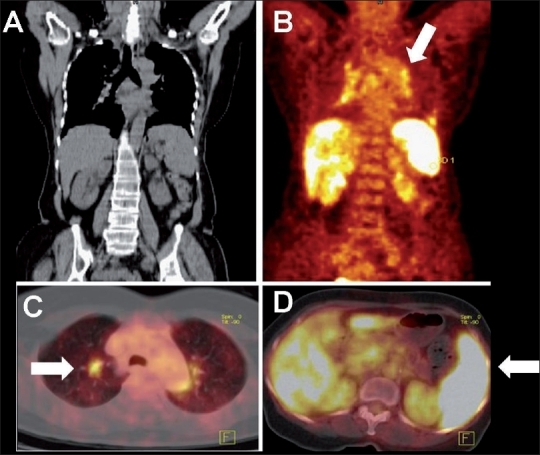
Disseminated TB with multifocal hepatic and diffuse splenic uptake. Coronal plain CT (A) and PET (B) with axial PET/CT (C, D) images in a patient with FUO show diffuse increased FDG uptake in an enlarged spleen and multifocal uptake in the liver, mediastinal nodes, and patchy lung lesions (arrows). Transbronchial sampling from the subcarinal nodes revealed tuberculosis. PET in this case guided the tissue sampling from an active lesion in an accessible site, using the least invasive route
